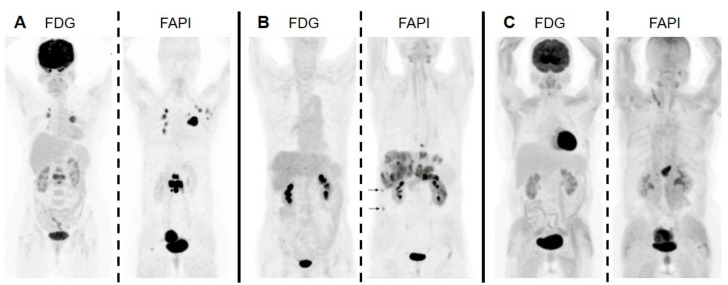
Cases in which FAP-targeted imaging outperformed [^18^F]FDG PET/CT. (A) Invasive lymphnodal metastatic duct breast carcinoma. (B) Pancreatic duct adenocarcinoma with liver metastases. (C) Poorly differentiated gastric adenocarcinoma. Image sections were taken from Kömek et al., Chen et al. and Kuten et al.; copyright Springer-Verlag GmbH Germany, part of Springer Nature [38,39,40].
